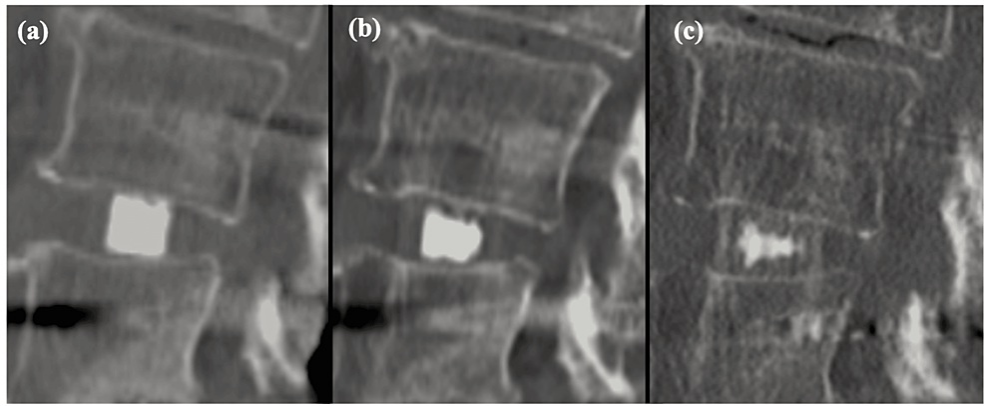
Progression of interbody fusion on sagittal CT scans at (a) seven days, (b) one year, and (c) two years postoperative follow-up. Bone fusion was not obtained one year after the surgery, but bone fusion was obtained two years later.
